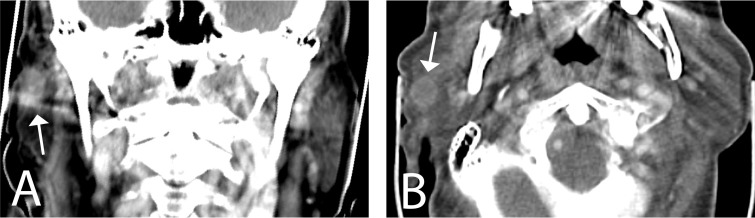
Representative face contrast-enhanced CT slices Coronal (A) and axial (B) reconstructions showing a single slightly heterogeneous, well circumscribed enhancing nodular lesion within superficial middle third (arrow) of right parotid gland characterized by a thin fibrous capsule surrounding the lesion.
